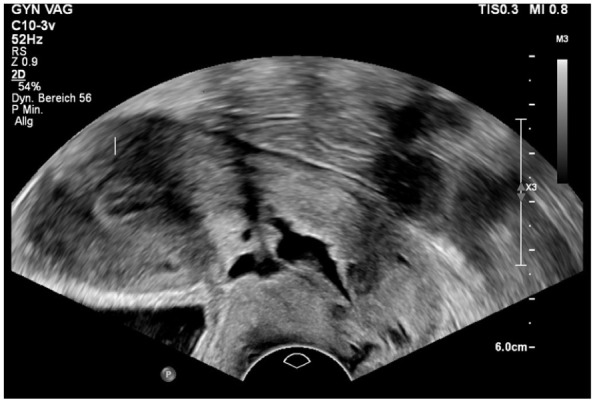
Transvaginal sonographic finding of a complex isthmocele with multiple branching tracts extending from the caesarean scar site.
